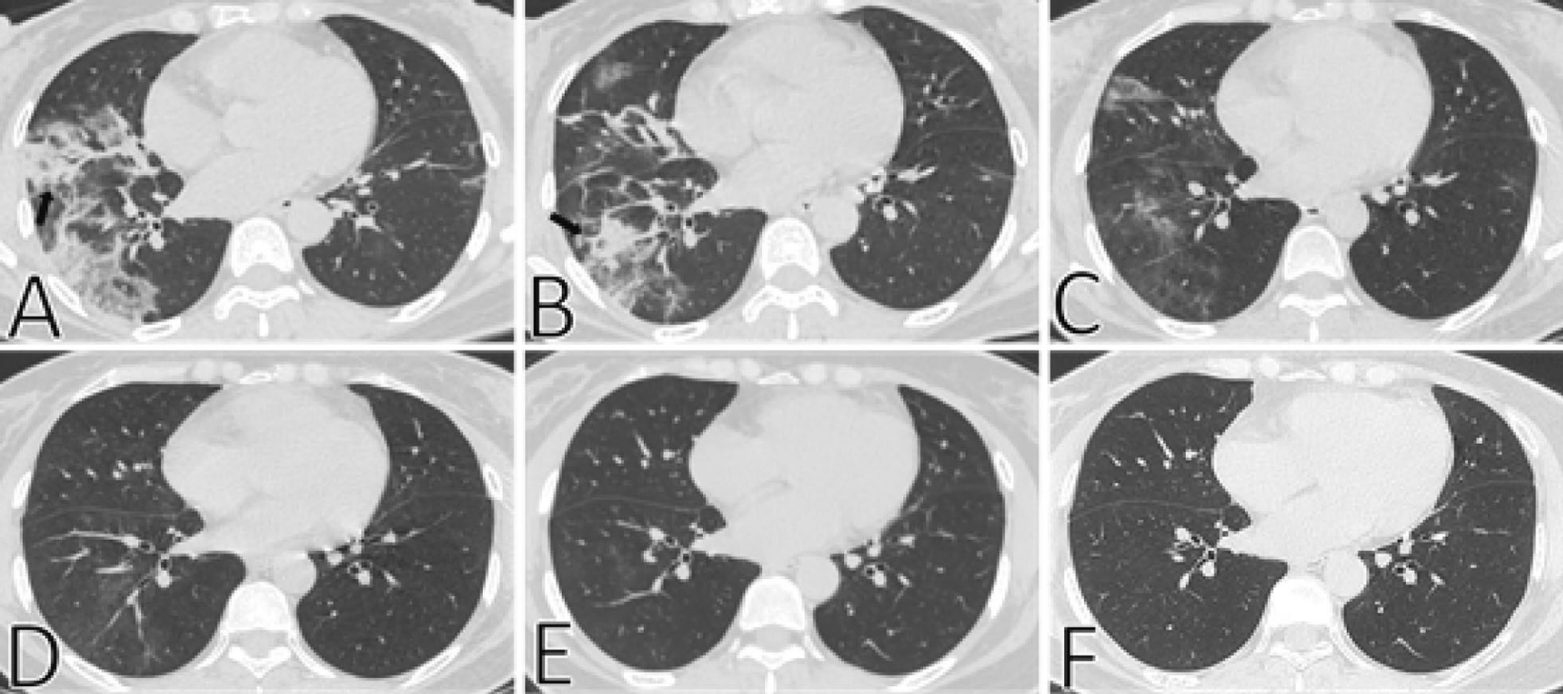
Typical CT imaging findings of a 53-year-old woman with resolved pulmonary fibrosis. A Thin-section chest CT scan in our hospital on February 7, 2020 (4 days after symptoms onset). Chest CT imaging showed multiple lesions in both lungs, a mass of ground-glass opacities was observed in the middle and lower lobes of the right lung, with consolidation and irregular interfaces (black arrow). B On February 9, 2020 (6 days after symptoms onset), the resolution of the lesion was obvious, ground-glass opacities, consolidation and irregular interfaces (black arrow) were still observed. C On February 12, 2020 (9 days after symptoms onset), the lung lesions were further resolved, the density of consolidation decreased. D, E On February 22, 2020 (19 days after symptoms onset) and March 14, 2020 (40 days after symptoms onset), respectively, only a little ground-glass opacities were observed in the lower lobe of the right lung, with obscure boundaries. F On May 22, 2020 (108 days after symptoms onset), the lesions in both lungs have been completely resolved
